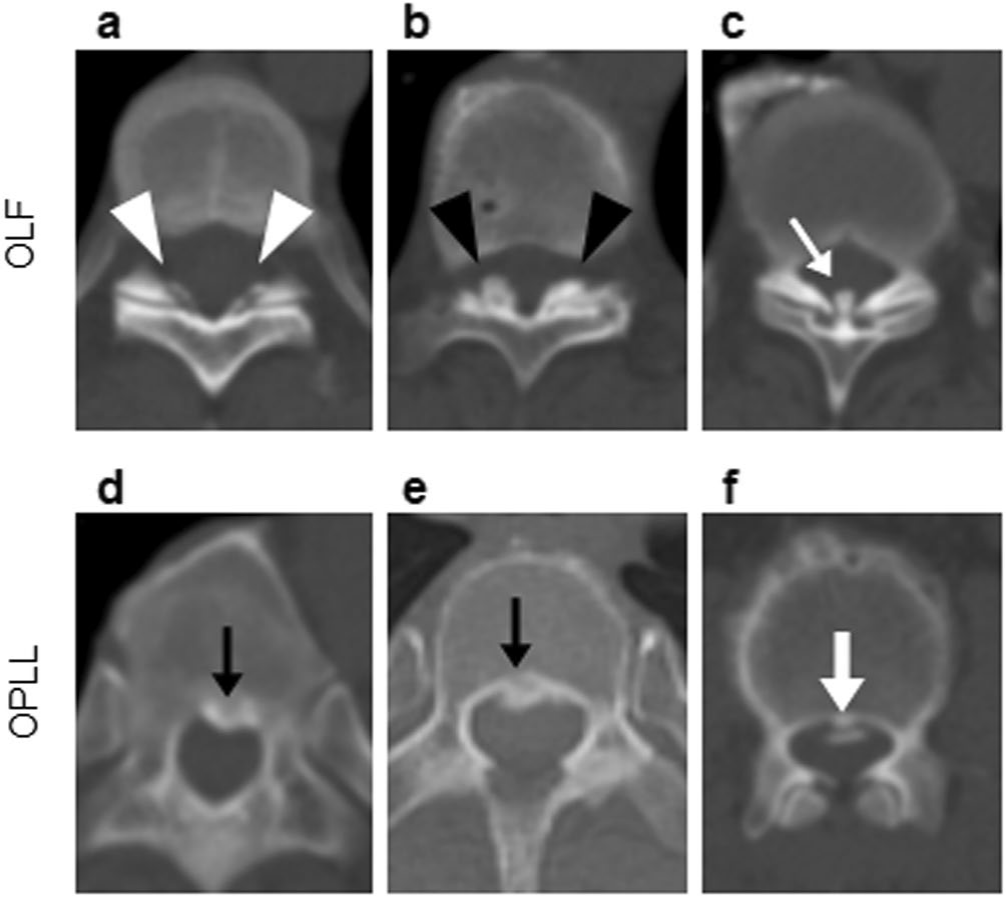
Criteria for the identification of OLF and OPLL on computed tomography. (a) OLF that is clearly visible but less than 3 mm in thickness (white arrowheads). (b) OLF with an apparent thickness of 3 mm or more (black arrowheads). (c) A mushroom-shaped OLF that is localized at the center of the laminae (white arrow). (d,e) OPLL with an apparent thickness of 2 mm or more (black arrow). (f) OPLL that is small but with a thickness of 2 mm or more (bold white arrow). OLF, ossification of the ligamentum flavum; OPLL, ossification of the posterior longitudinal ligament.
